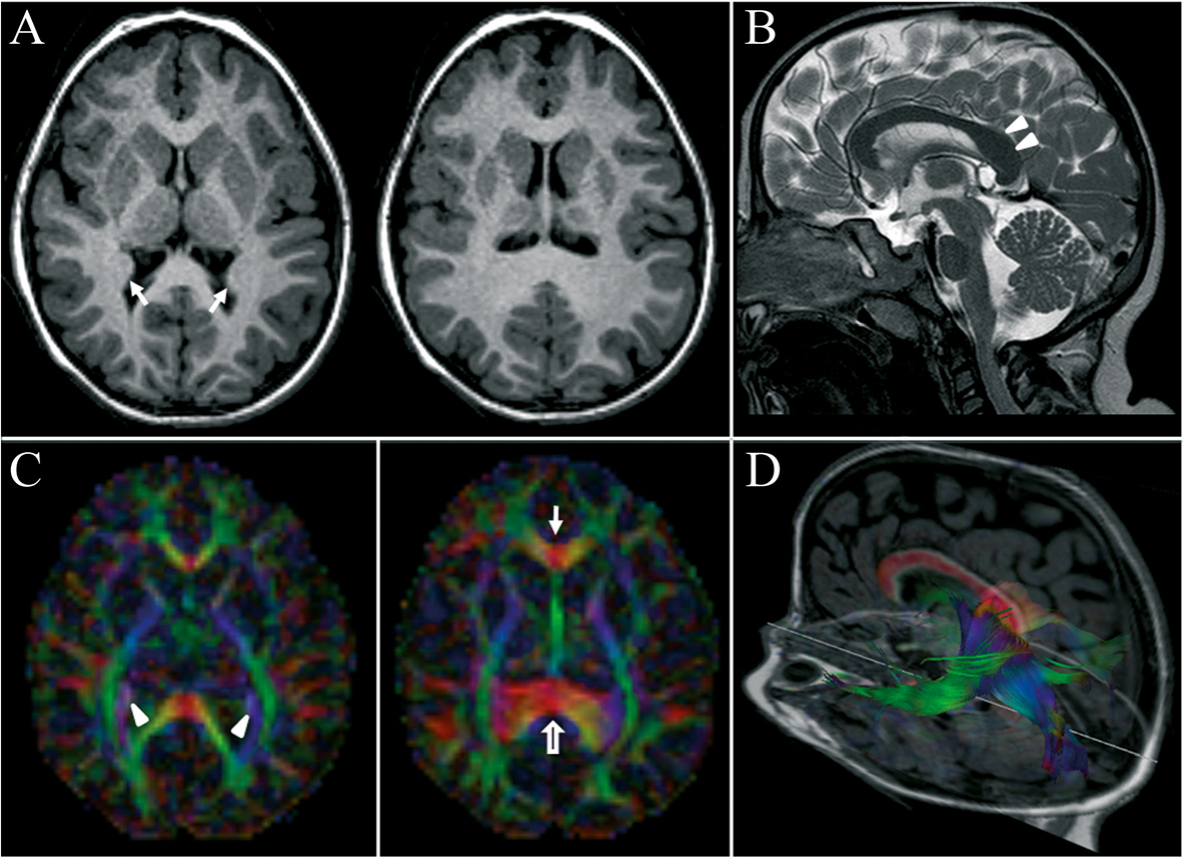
Brain MRI and DTI at 2 years of age. (A) Axial T1-weighted images reveal microcephaly with simplified cortical gyration and underdevelopment of the frontal lobes. Note the inward deformation of the lateral walls of the trigones (arrows). (B) Sagittal T2-weighted image shows pontine hypoplasia and dysmorphic corpus callosum with enlarged splenium (arrowheads). (C) Corresponding axial fractional anisotropy (FA)-weighted colour directional maps confirm the disproportion between the genu (arrow) and the splenium (empty arrow) of the corpus callosum. Note the presence of abnormally thickened fiber bundles with superior-to-inferior direction, located between the optic radiations and the lateral ventricles (arrowheads). The fibers are color-coded by direction: red is left-to-right (and vice versa), green is anterior-to-posterior (and vice versa), blue is superior-to-inferior (and vice versa). (D) DTT, oblique view, shows the course of the splenium of the corpus callosum superimposed on sagittal and axial T1-weighted images fused with FA-weighted colour directional map. The inward deformation of the lateral walls of the trigones is due to an abnormally thick tapetum. The tapetum is made of fibers of the corpus callosum connecting the inferior temporal lobes.
